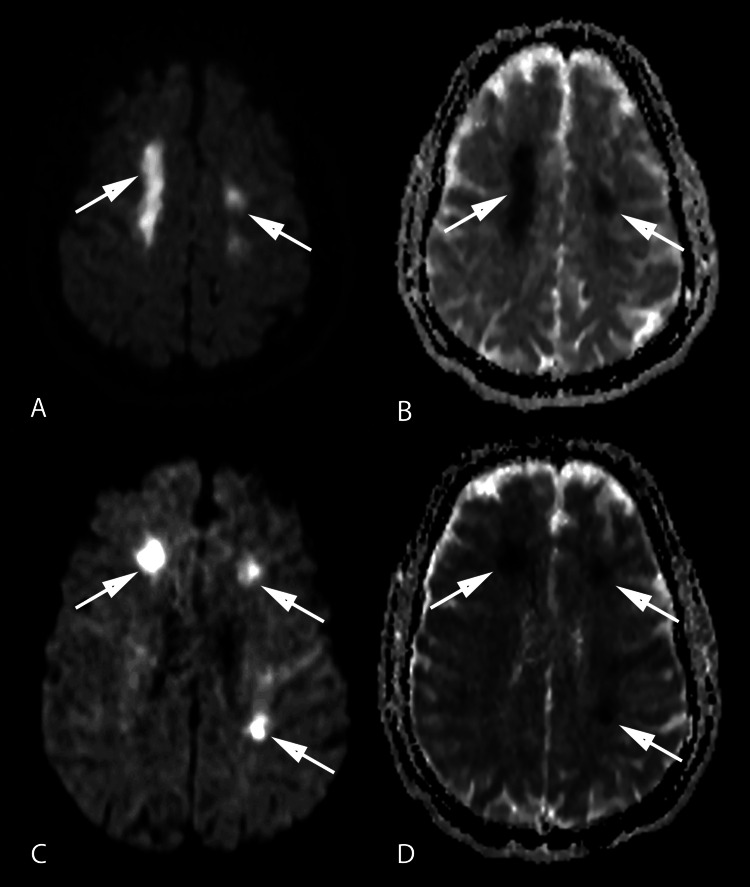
MRI Brain showing acute ischemic infarcts A and C: Axial DWI images of the brain showing multiple areas of diffusion restriction in bilateral centrum semiovale extending up to frontal periventricular white matter; B and D: Axial ADC images of the brain showing areas of low ADC values in bilateral centrum semiovale and periventricular white matter suggesting acute infarcts MRI = Magnetic Resonance Imaging; DWI = Diffusion-Weighted Imaging; ADC = Apparent Diffusion Coefficient
